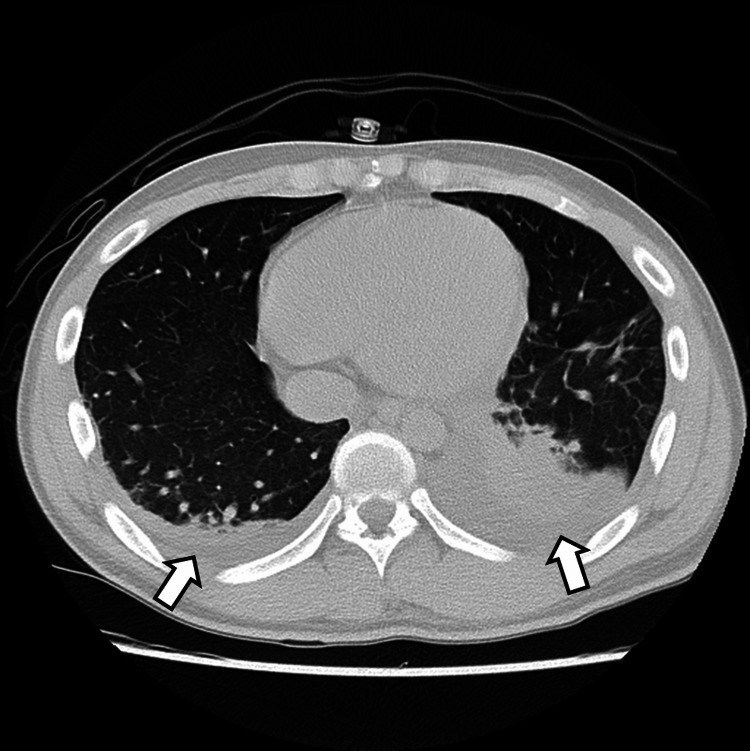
A CT image of the chest on day nine revealed pleural effusion and atelectasis.
